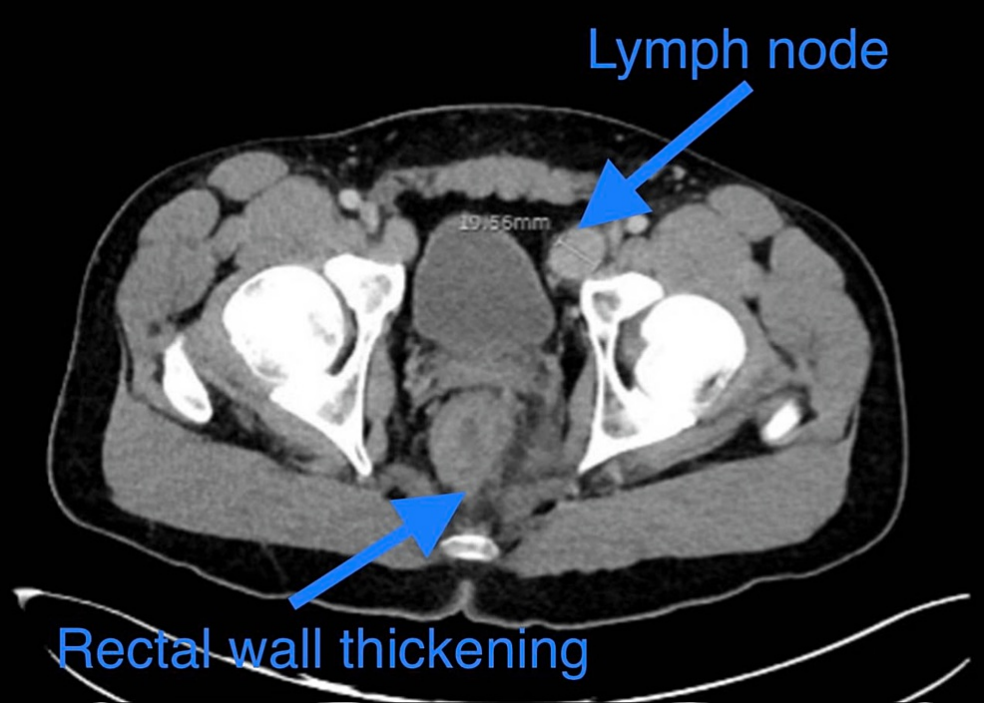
CT abdomen and pelvis depicting rectal thickening and an enlarged lymph node. CT, computed tomography
